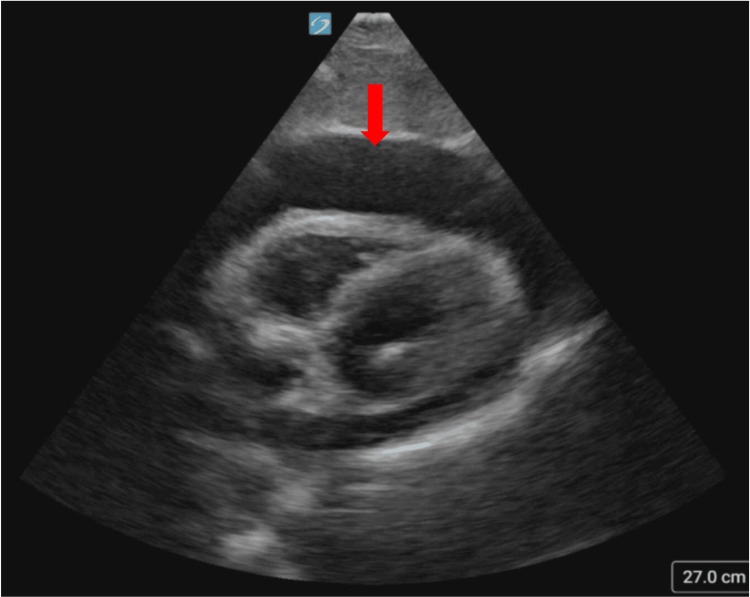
Point-of-care ultrasound on admission, with the arrow indicating the large pericardial effusion
